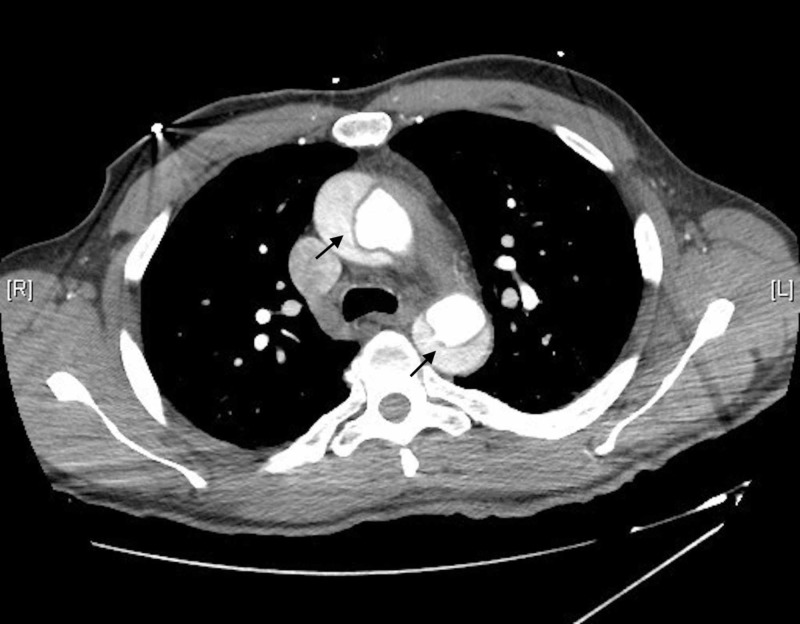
CT image of intimal flaps in ascending and descending aorta CT, computed tomography
